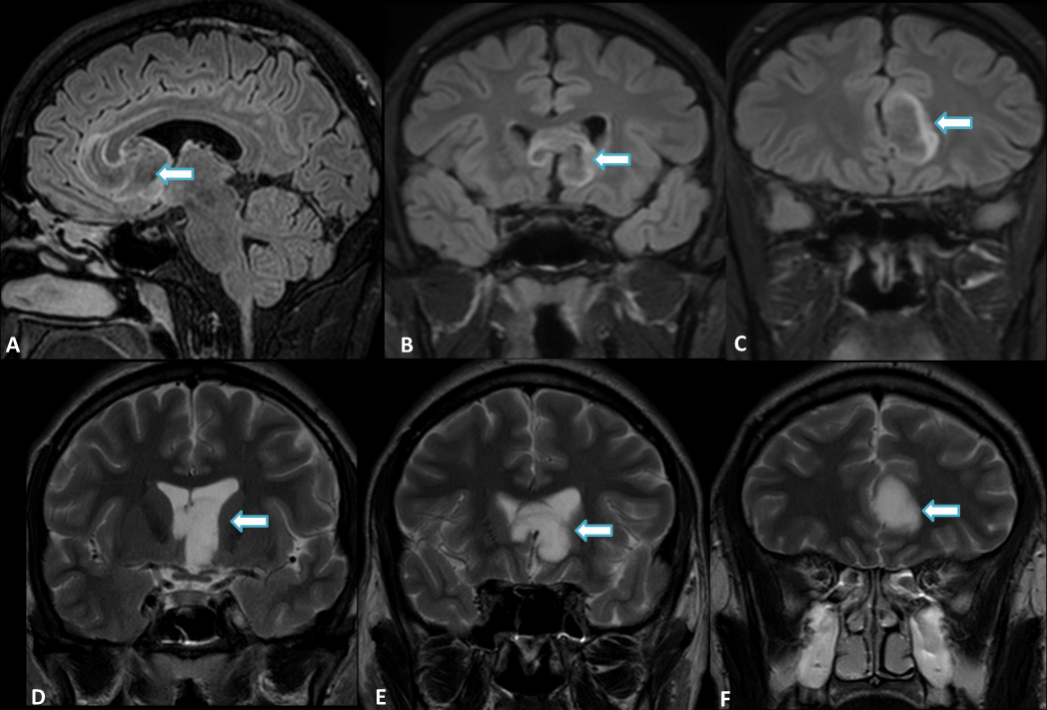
Sagittal and coronal FLAIR (A, B, C) and T2WI (D, E, F) of the same patient better characterize the large infiltrative lesion (arrows) in the left septal area (subcallosal gyrus) involving the genu of the corpus callosum, with anteroinferior extension to the septum pellucidum, left frontal horn of the lateral ventricle and ipsilateral III ventricle. The partial suppression and hyperintense rim are also shown on FLAIR. Note the “L” shaped appearance on Coronal images.
